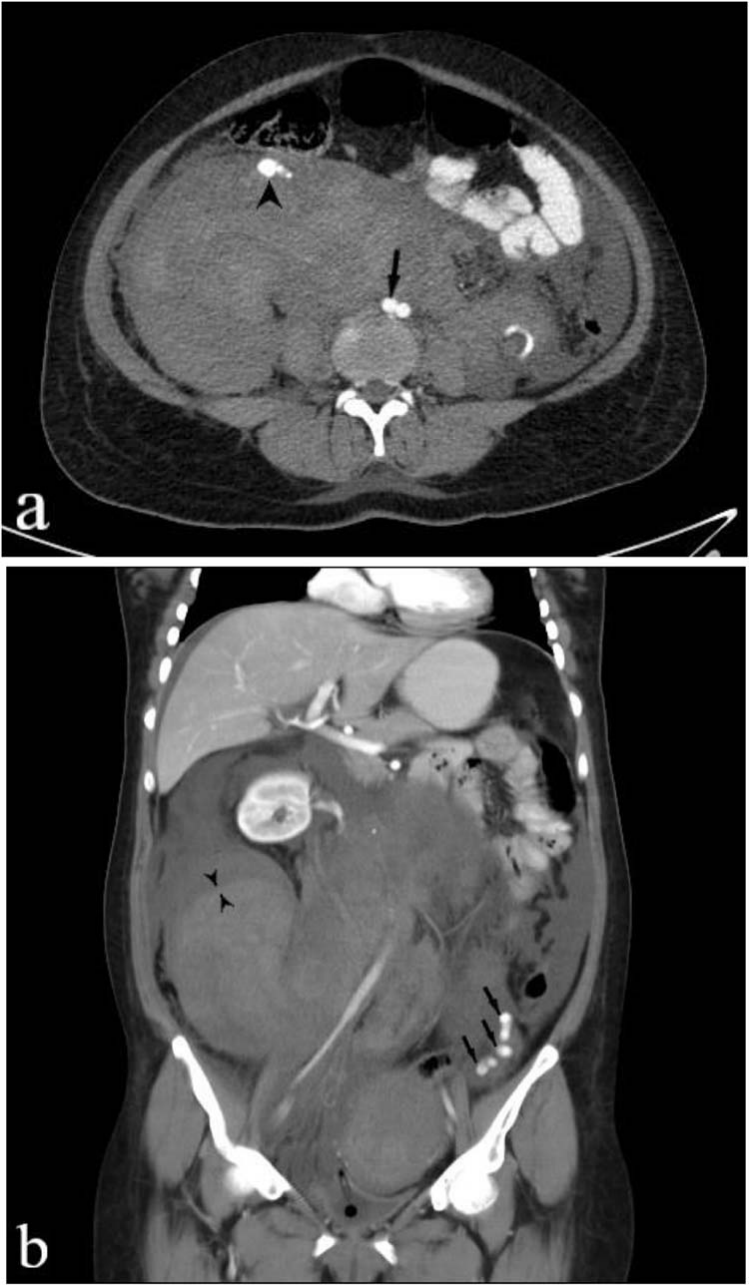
IV and PO contrast-enhanced CT in portal phase shows a massive heterogeneous hematoma in the pelvic region, dominantly in the right lower quadrant. (a) An axial section at the level of abdominal aortic bifurcation, shows the dilated and anteriorly displaced right ovarian artery (arrowhead), indicating an aneurysm—its size is comparable to the common iliac artery (arrow). (b) A coronal reconstruction of the retroperitoneum demonstrates the extent of hematoma. The left ovarian artery manifests multiple aneurysms (arrows), and the obvious border between two regions of hematoma with different densities (paired arrowheads) shows separate bleeding episodes.
